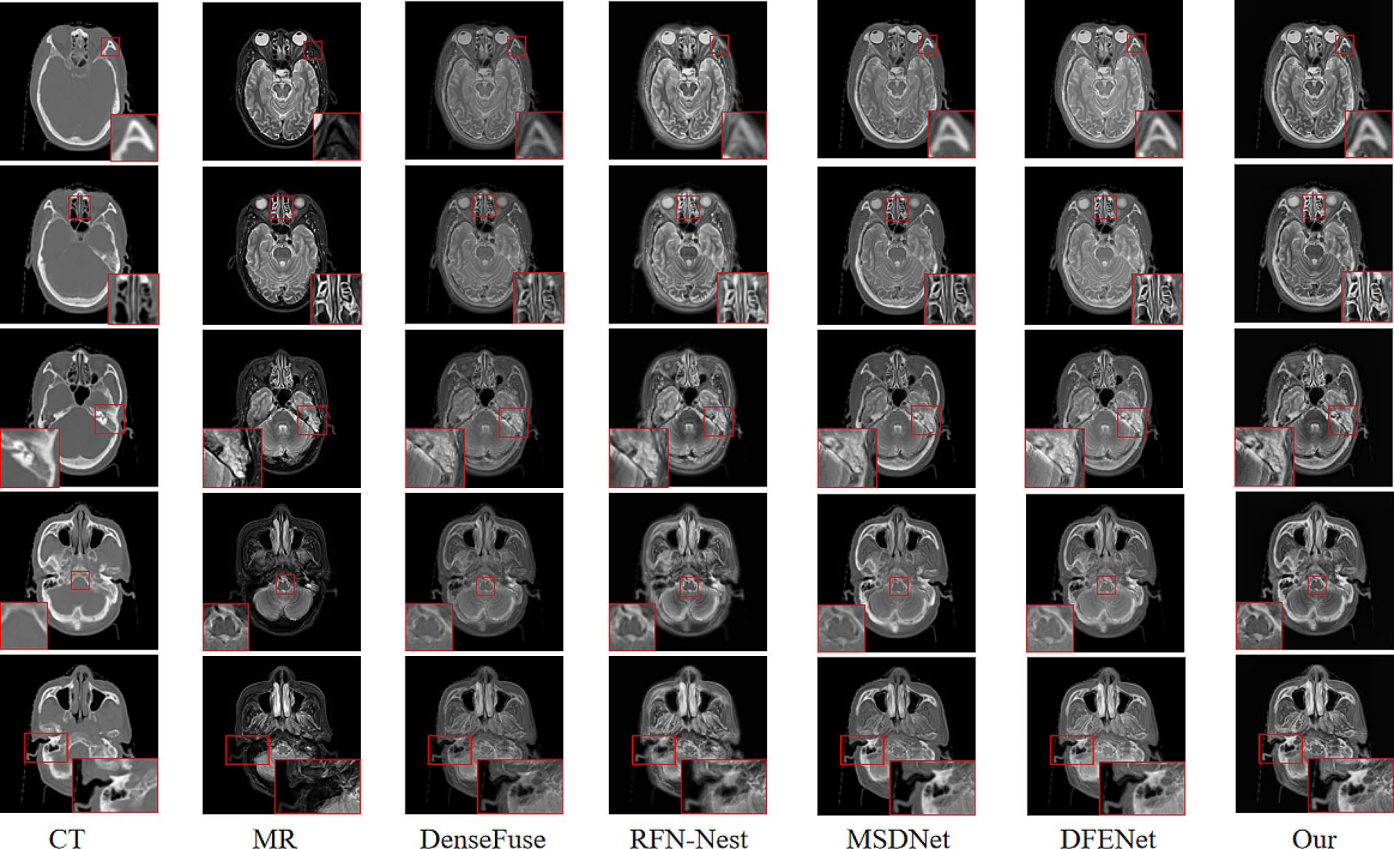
Qualitative comparison: CT and MR images of the first and second columns. The following three columns show the fusion results of the comparison method and the method proposed in this article
